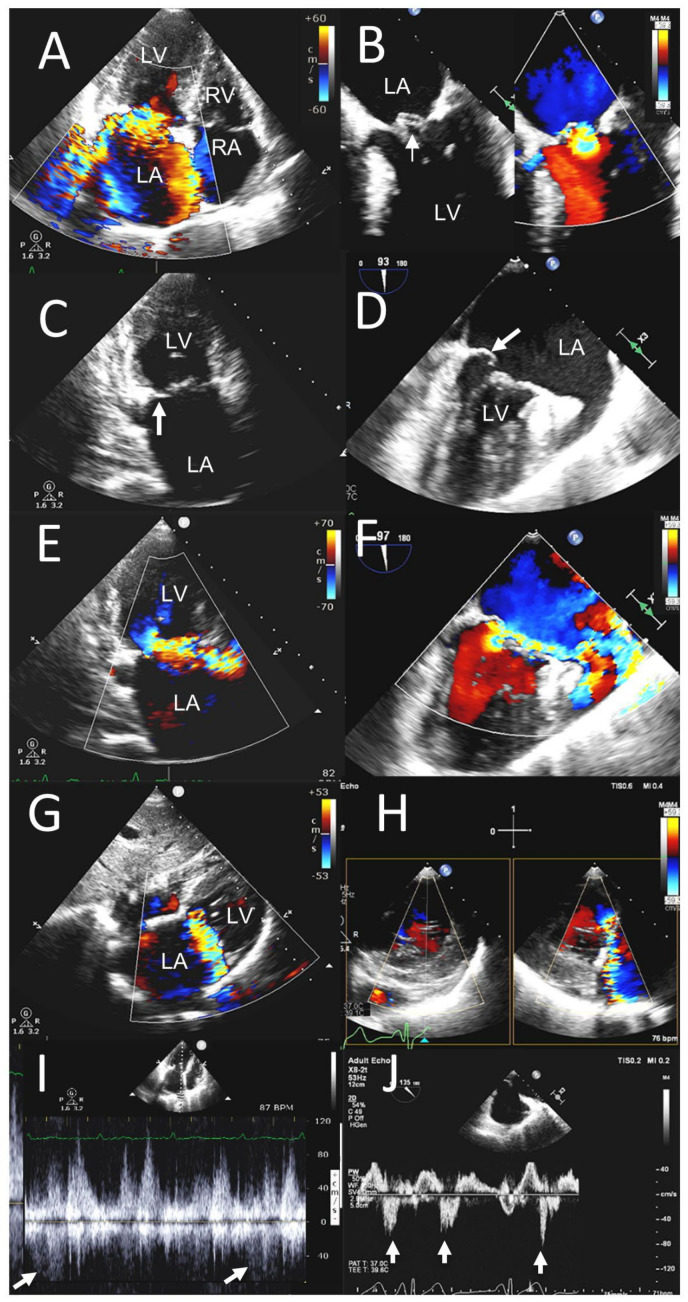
POCUS in assessment of MR severity and mechanism. An 86-year-old female was seen for a cardiac murmur and an episode of possible atrial arrhythmia. POCUS images (left-side panels) are shown compared to the TEE images (right-side panels). (A,B) Four-chamber views showing severe MR, with MR jet originating from medial commissural region and curling around the anterolateral LA wall (A), a flail A3 segment and proximal isovelocity surface acceleration (PISA) at a normal aliasing velocity of 59.3 cm/s (B). Severe LA enlargement is shown in both views. (C,D) Two-chamber views showing A3 prolapse (white arrow (C)) and flail A3 segment (white arrow (D)) along with dilated LA in (C,D). (E,F) Two-chamber color Doppler views showing an eccentric MR jet originating from medial commissural region and entering the LA appendage at aliasing velocities of 79 and 59.3 cm/s, respectively. (G) Subcostal four-chamber views showing MR jet originating from medial commissural region. (H) TEE biplane view showing PISA of MR jet from medial commissure at a normal aliasing velocity of 59.3 cm/s. (I) Apical four-chamber view with PW Doppler in the right upper pulmonary vein. (J) Mid esophageal view of the RA, interatrial septum and PW Doppler in the right upper pulmonary vein showing systolic flow reversal (white arrows (I,J)). LA, left atrium; LV, left ventricle; RA, right atrium; RV, right ventricle.
